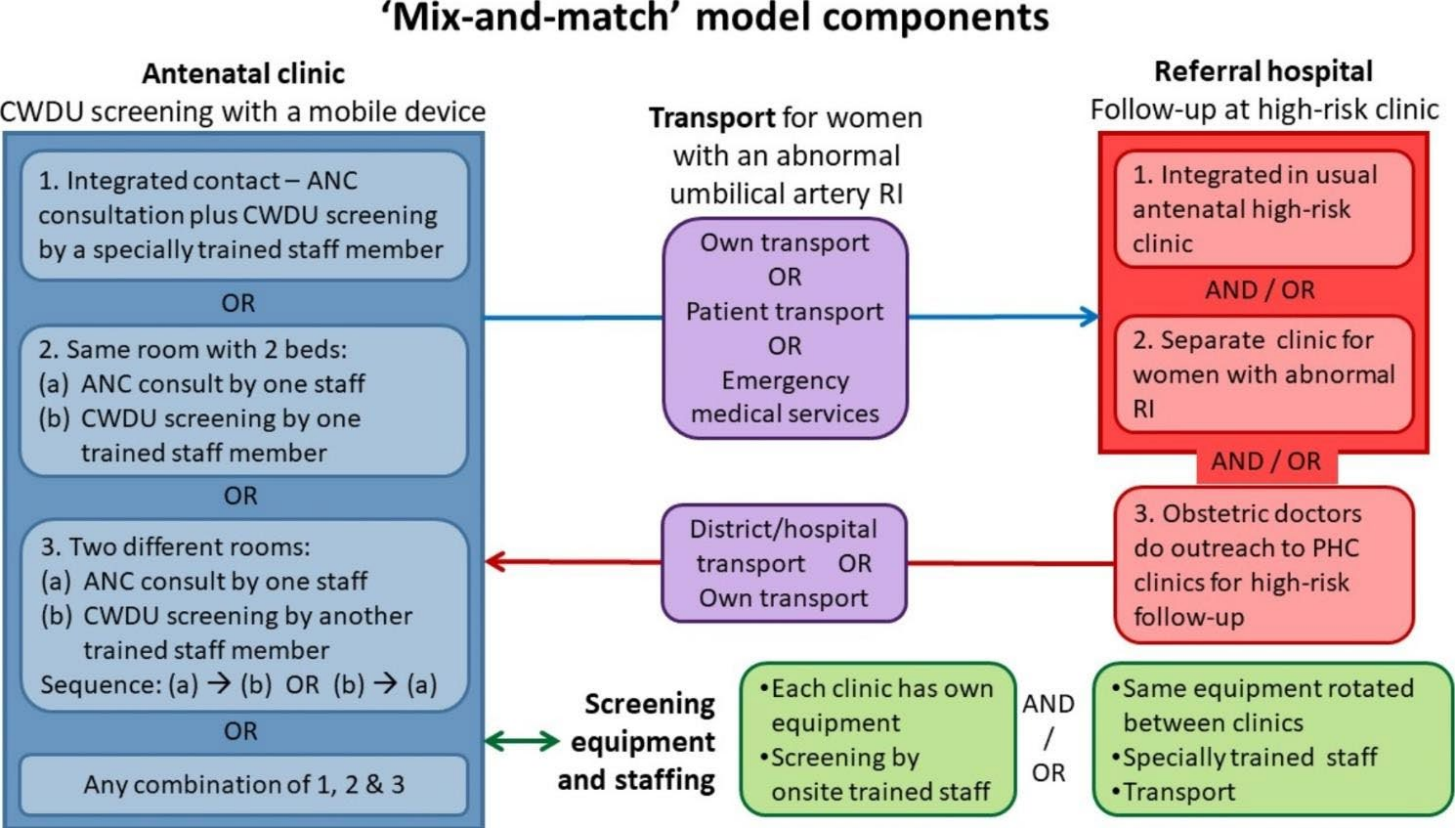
Components for building implementation models for CWDU screening CDWU, continuous-wave Doppler ultrasound; ANC, antenatal care; RI, resistance index; PHC, primary healthcare
